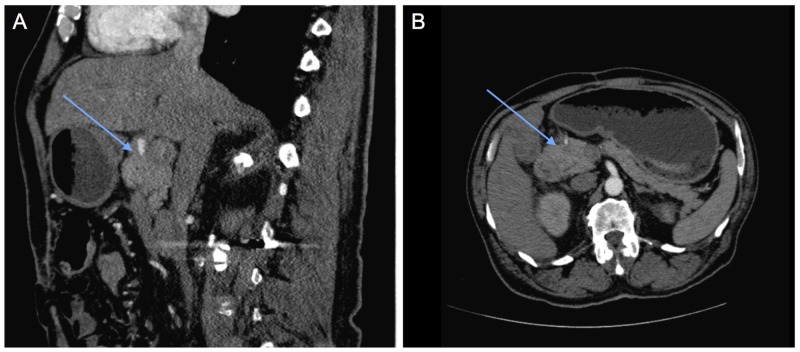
Triple phase computed tomography (CT) pancreas significant for abnormal 7.7 cm x 4.6 cm soft tissue mass encasing common hepatic artery and origin of gastroduodenal artery (as indicated by blue arrows), observable in both (A) sagittal and (B) transverse views.
